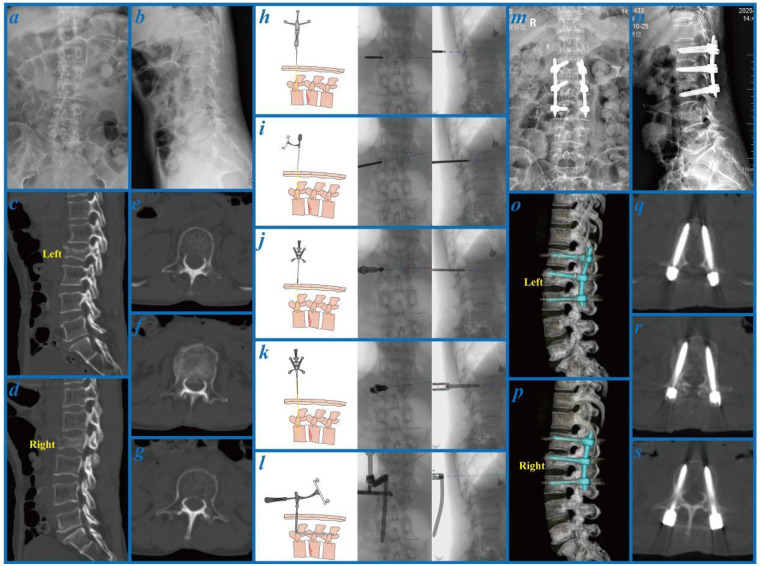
A 42-year-old female patient with an L2 burst fracture treated with PPSF under 2D navigation. (a,b) Preoperative anteroposterior and lateral radiographs showing the L2 burst fracture. (c–g) Preoperative sagittal and axial CT reconstructions demonstrating the L2 burst fracture with intact pedicles. (h) Navigated pedicle probe used to identify the optimal entry point and skin incision. (i) Navigated pedicle awl advanced into the pedicle and vertebral body along the planned trajectory. (j) Navigated pedicle tap employed to prepare the screw channel. (k) Under navigation guidance, a pedicle screw was inserted into the vertebral body using a navigated screwdriver. (l) A fixation rod of appropriate length was selected and positioned under navigation guidance. (m,n) Postoperative anteroposterior and lateral radiographs confirming satisfactory screw fixation. (o–s) Postoperative sagittal and axial CT images demonstrating accurate screw placement and adequate restoration of vertebral height.
